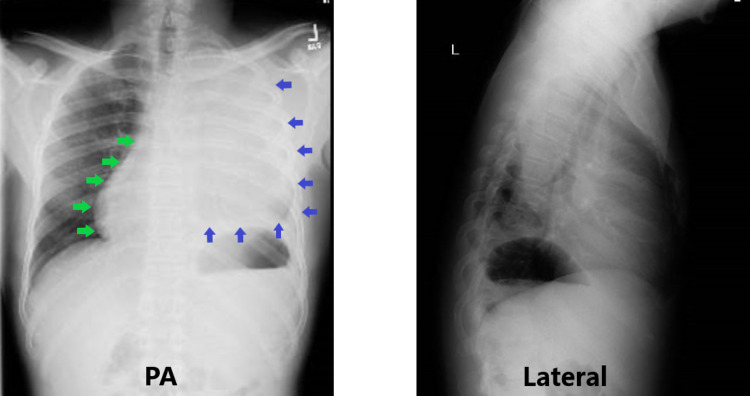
Posteroanterior (PA) and lateral chest x-ray. Complete opacification of the left hemithorax (blue arrows). Rightward cardiomediastinal shift (green arrows).
